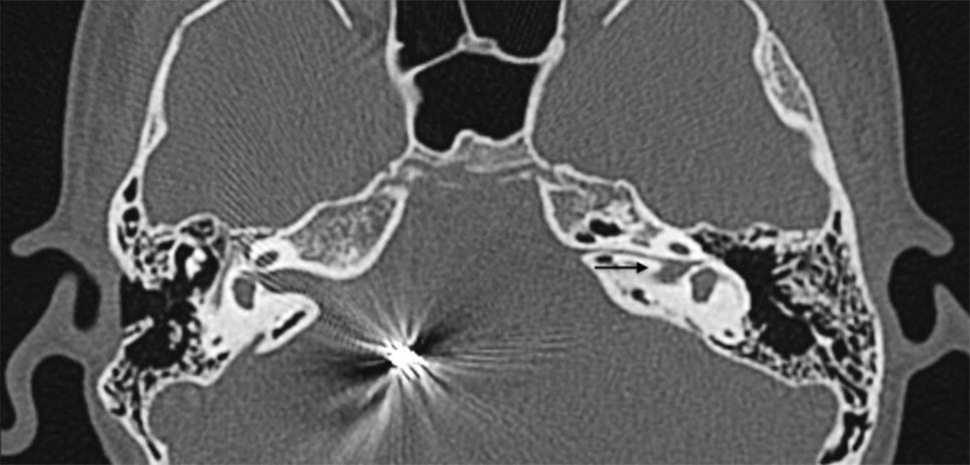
A 8-year-old female with bilateral complete HL since birth. Axial CT shows IAC partition on the left side characterised by an independent bony canal for the facial nerve that runs anteriorly (arrow) and an IAC that runs posteriorly (asterisk) populated by a hypoplastic cochlear nerve (not appreciable in CT imaging). Artefacts due to ABI on the right emi-pons in IP-II malformation of the same side are also appreciable
